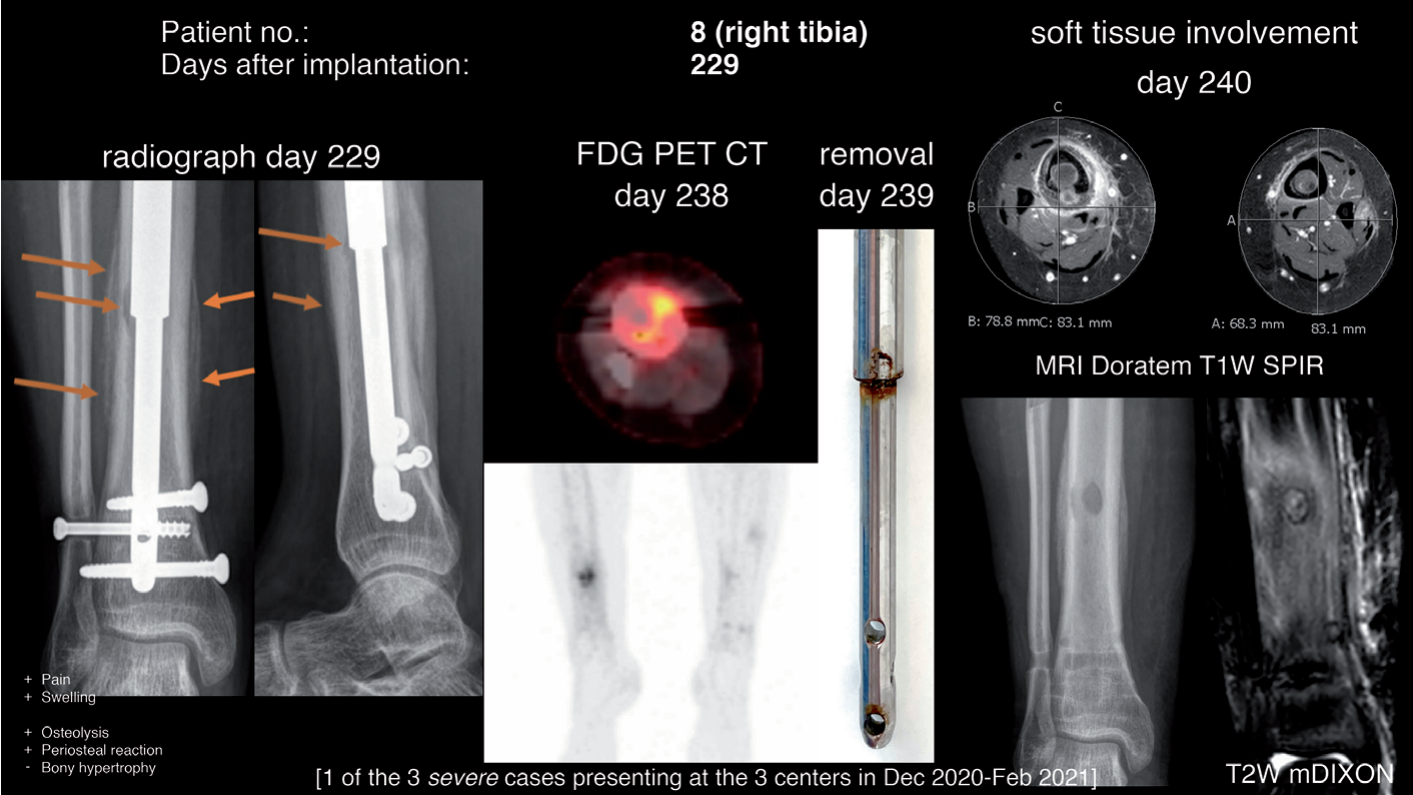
Osteolysis and periosteal reaction at postoperative day 229 (48 days after the onset of symptoms, i.e., pain in the area). FDG PET CT revealed increased glucose uptake in the area. The removed nail (day 239) had discoloration (potential corrosion) and on MRI on the subsequent day cortical destruction and periosteal reaction of the right tibia as well as soft tissue involvement were present. In comparison, a Stryde nail without discoloration was simultaneously removed from the asymptomatic left side.
